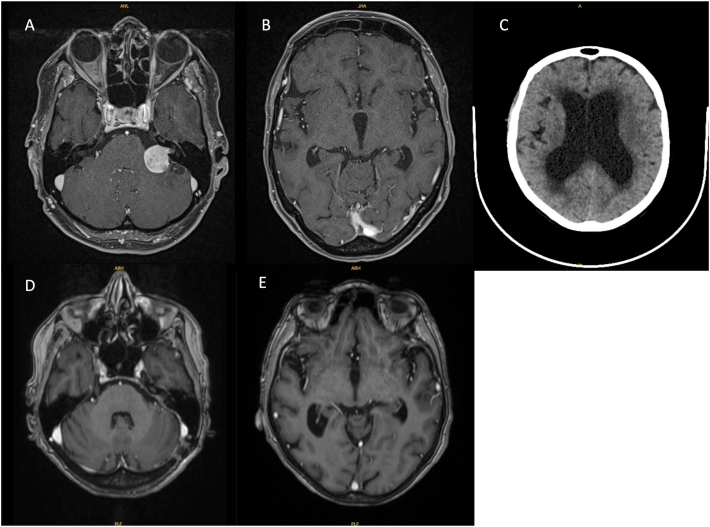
(A) Axial T1-weighted MRI showing a 2 cm left CPA tumor with (B) hydrocephalus. (C) Axial CT scan showing increased hydrocephalus at 48 h postoperatively treated by endoscopic third ventriculostomy. (D) Axial T1 weighted MRI showing total removal tumor and (E) regression of hydrocephalus.
